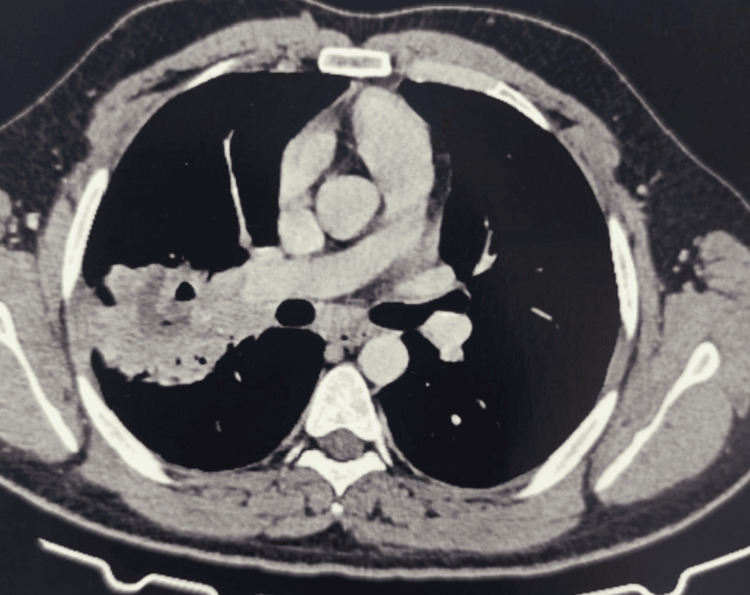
Chest computed tomography showing a cavitary consolidation Right lung mass extending from the apicodorsal segment of the right upper lobe to the middle lobe and toward the Fowler segment, heterogeneous in density, with necrotic content and associated intracavitary gas bubbles, featuring a thick wall with irregular margins. The mass demonstrates heterogeneous enhancement after contrast administration, delineating central necrotic areas measuring 70 × 40 mm.
